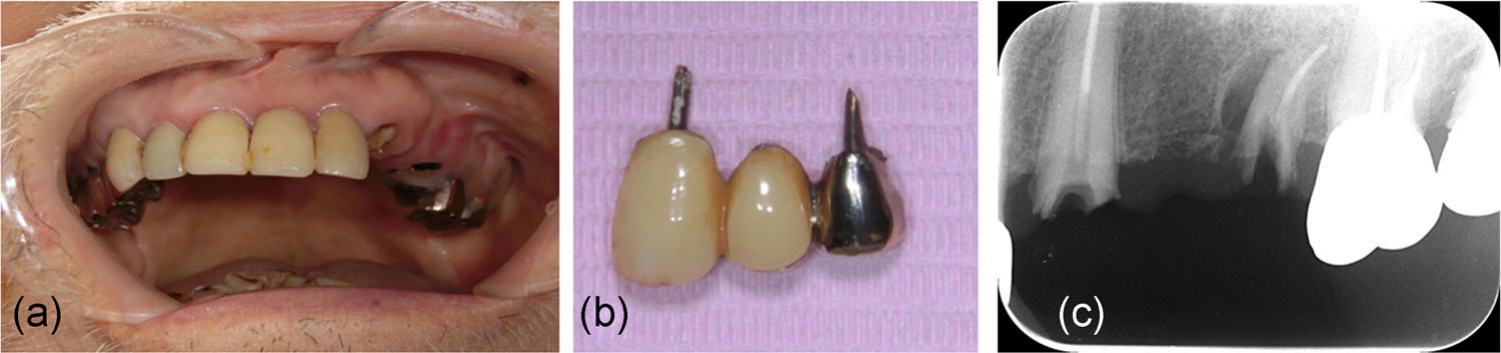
Oral findings in Case 2; (a) intraoral view of the upper jaw, (b) detached bridge, (c) intraoral radiograph
